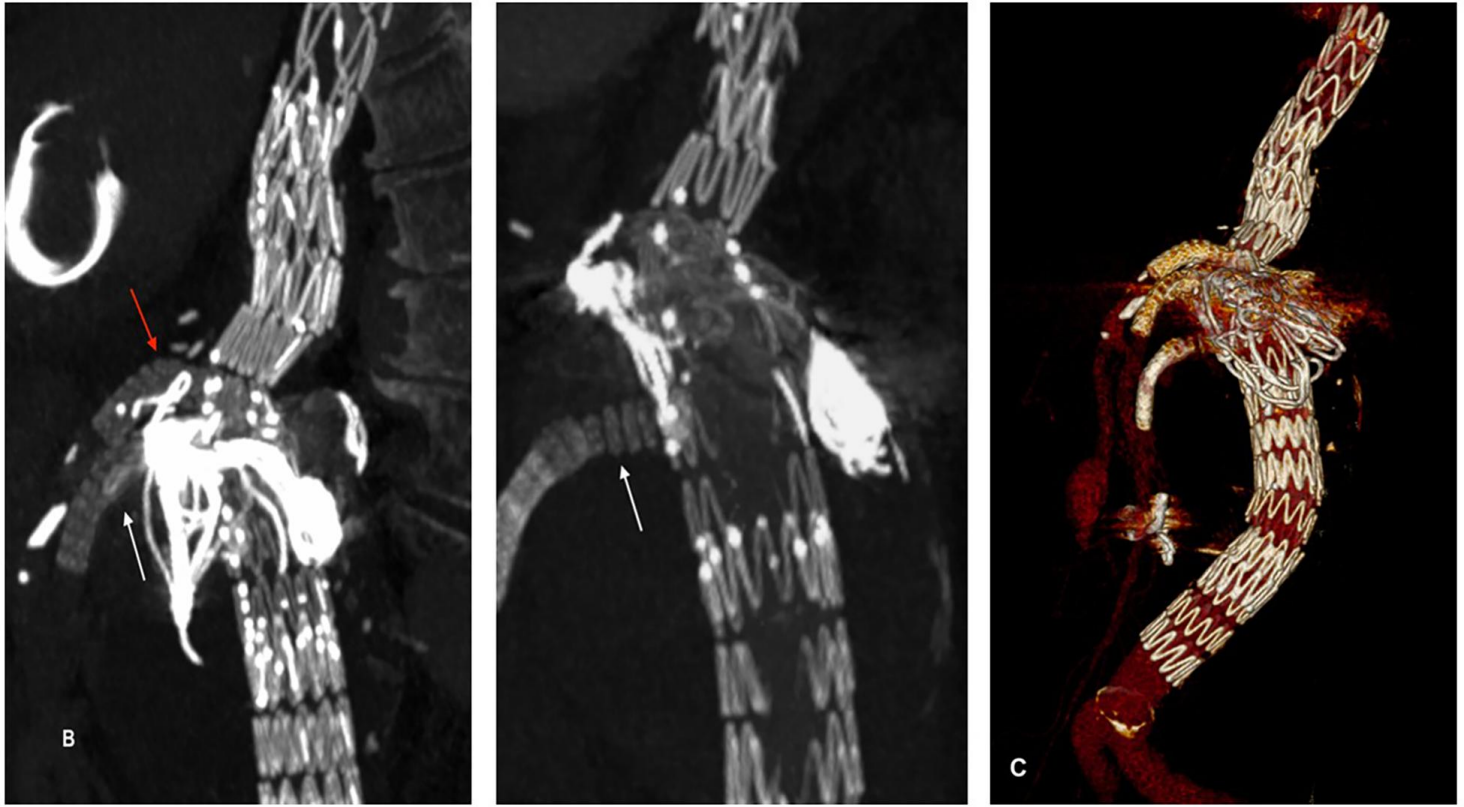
3D reconstructions of the 12-month CTA showing correct positioning and patency of the stents in the RRA, SMA, and CT, with no evidence of endoleaks. (A) relining of the RRA stent (white arrow). (B) relining of the SMA stent (white arrow) and placement of the stent in the CT (red arrow). (C) volume rendering. CT, celiac trunk; SMA, superior mesenteric artery; RRA, right renal artery.
